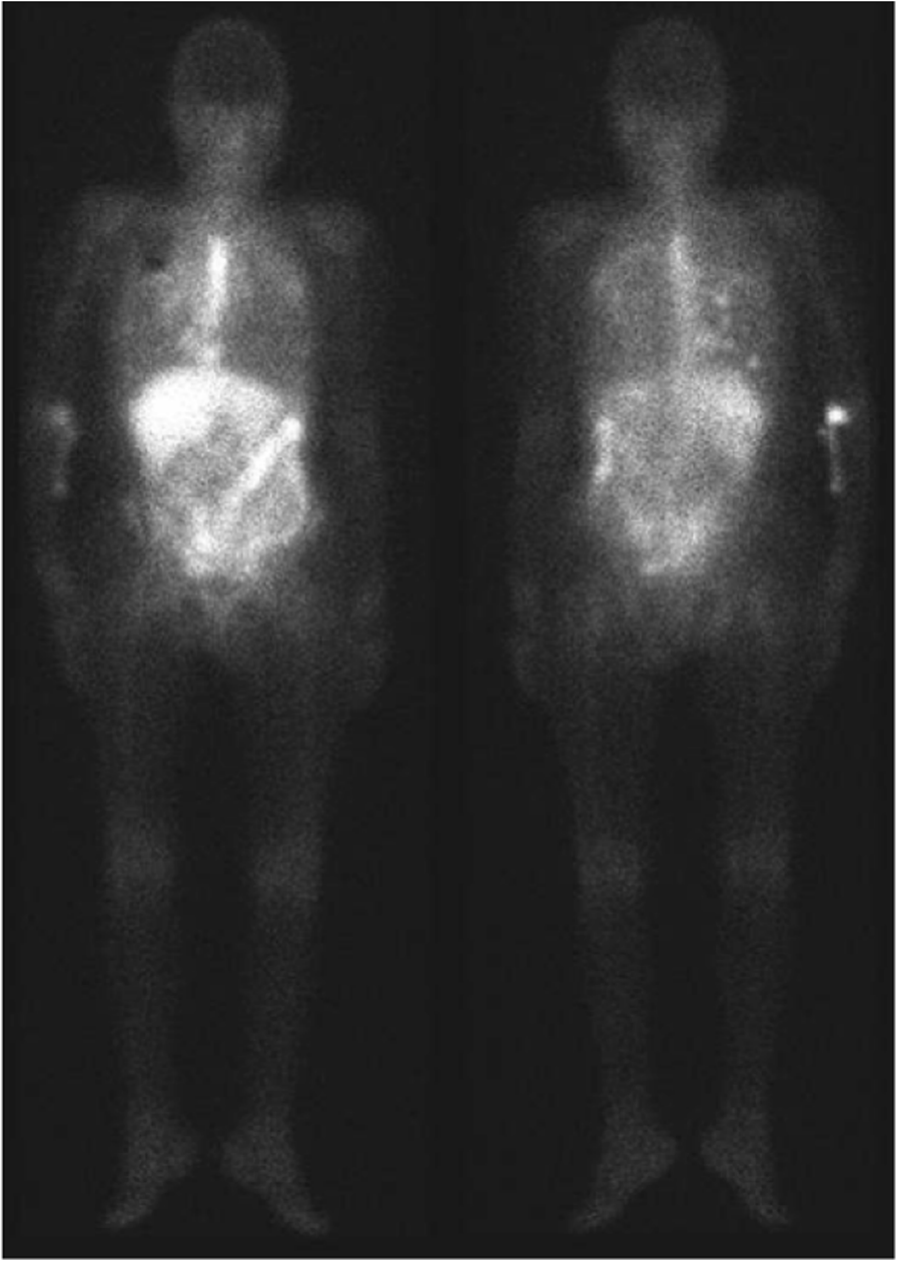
Gallium scintigraphy did not reveal any suspicious lesions expect the right lung mass
